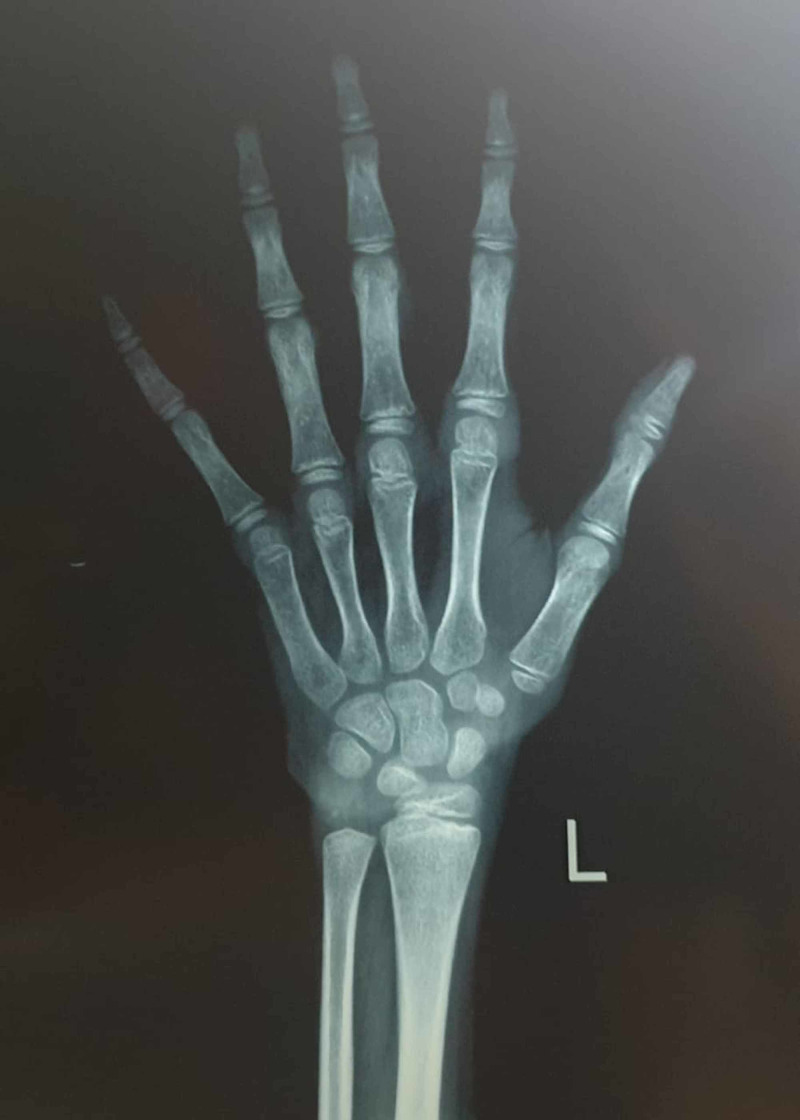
X-Ray of wrist to assess bone age of the patient. The bone age of trapezium, trapezoid, and scaphoid appears to be six years and the bone age of the distal epiphysis of the ulna seems to be five to eight years. That makes approximate bone age to be around seven to eight years. The chronological age of the patient is 18 years.
